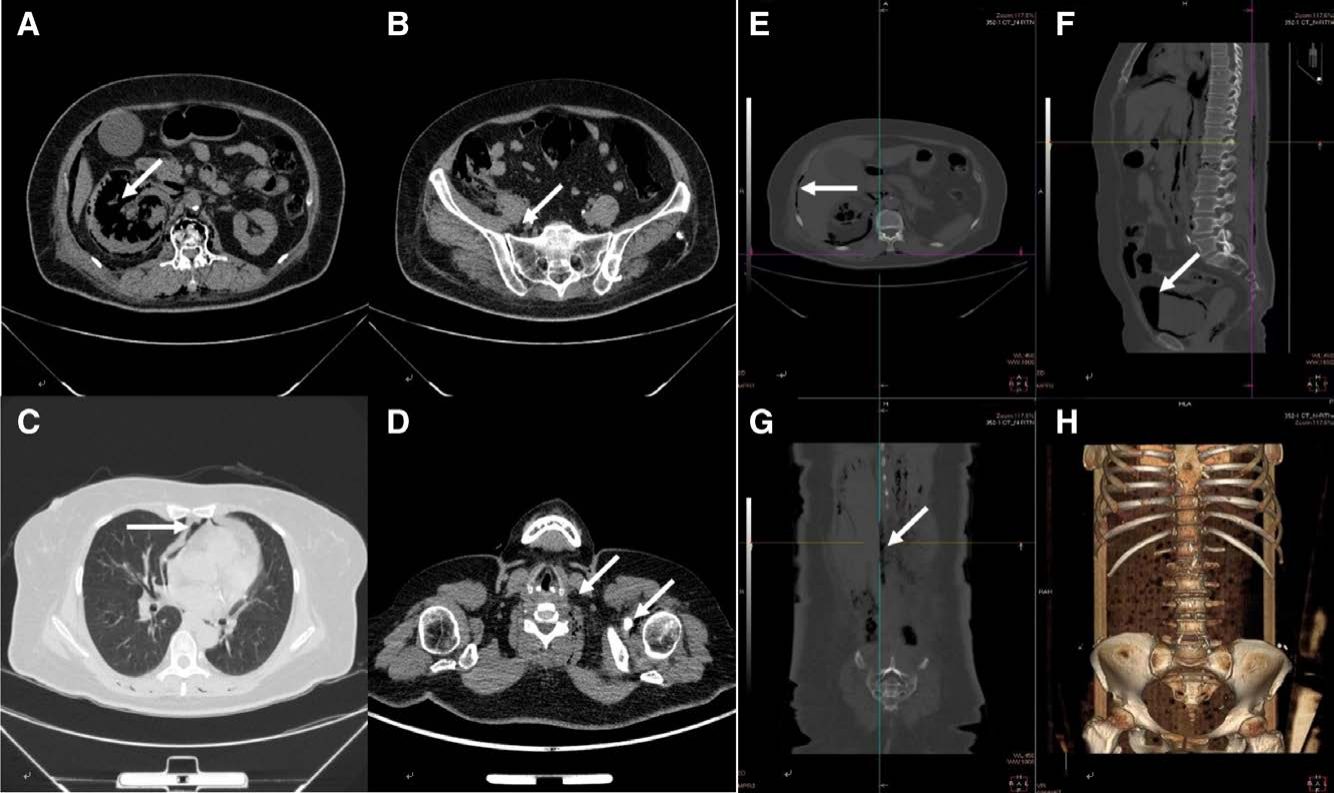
Extensive gas accumulations. Non-contrast-enhanced computed tomography scan shows the right renal parenchymal necrosis, with extensive perinephric gas accumulation (A), extending to involve the right iliac fossa (B), mediastinum (C), left shoulder and left side of the neck (D), perihepatic space (E), bladder (F), bilateral psoas and erector spinae muscles (G), and Extensive pneumoperitoneum and pneumopelvis are identified on imaging (H).
